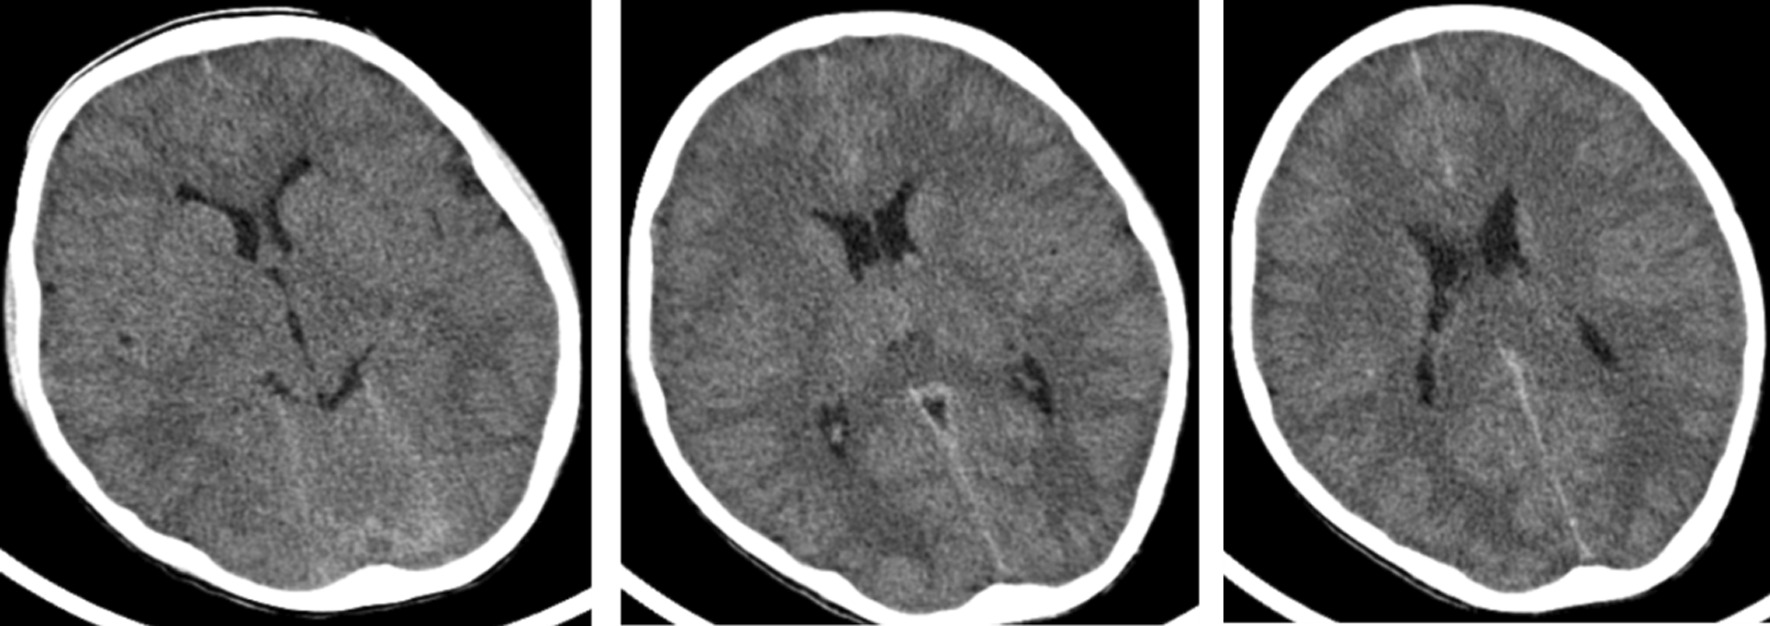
Patient cranium CT scans. Local density of cerebral sickle and tentorium slightly increased, subarachnoid hemorrhage was not excluded
